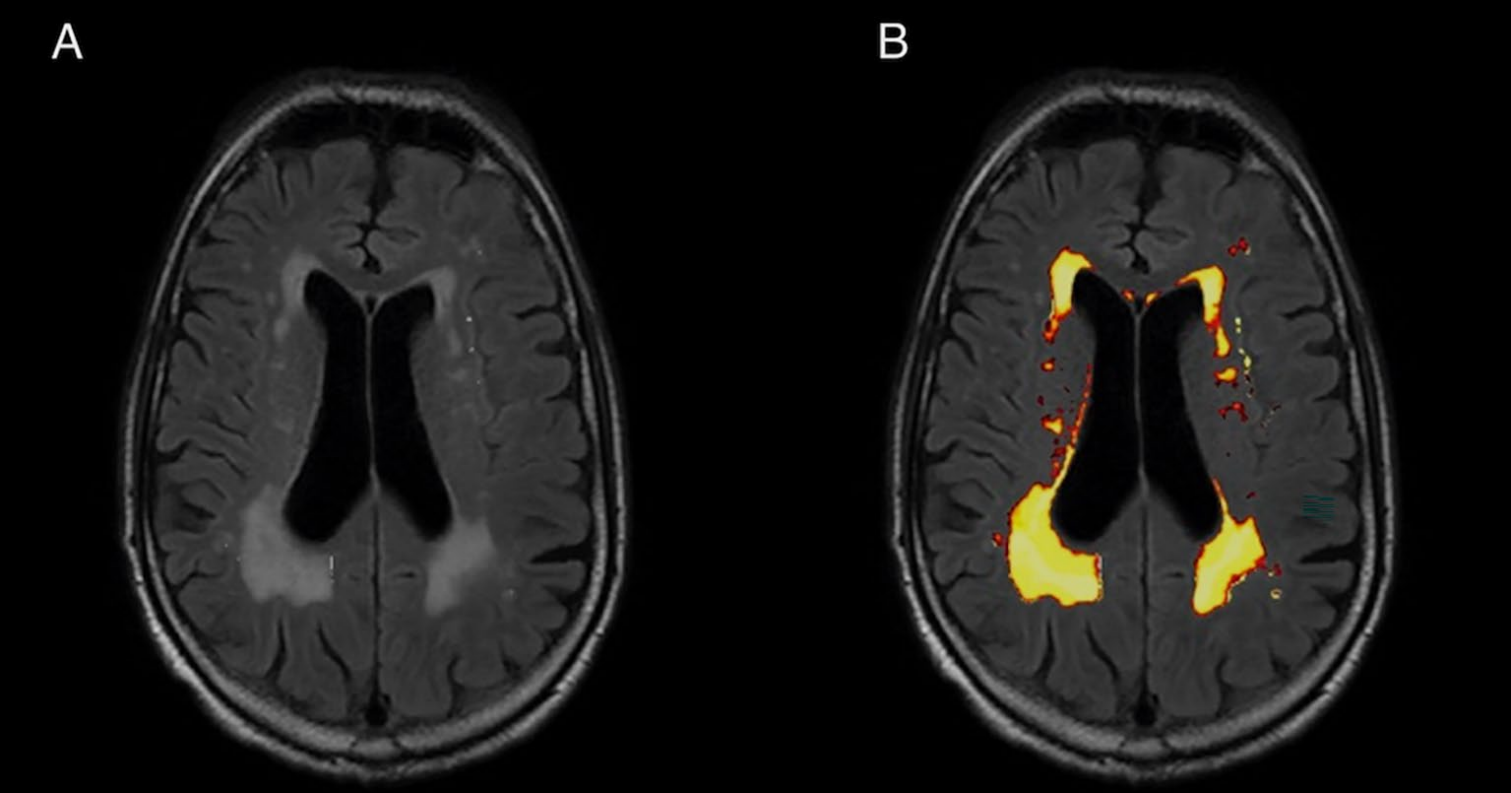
T2 FLAIR images from a 75-year old participant with hyperintense lesions (Fazekas 3) due to cerebral small vessel disease. (A) T2 FLAIR image showing multiple hyperintense white matter lesions. (B) Results of the white matter lesions segmentation using the lesion prediction algorithm. The white matter lesions detected by the algorithm are presented in red-yellow.
